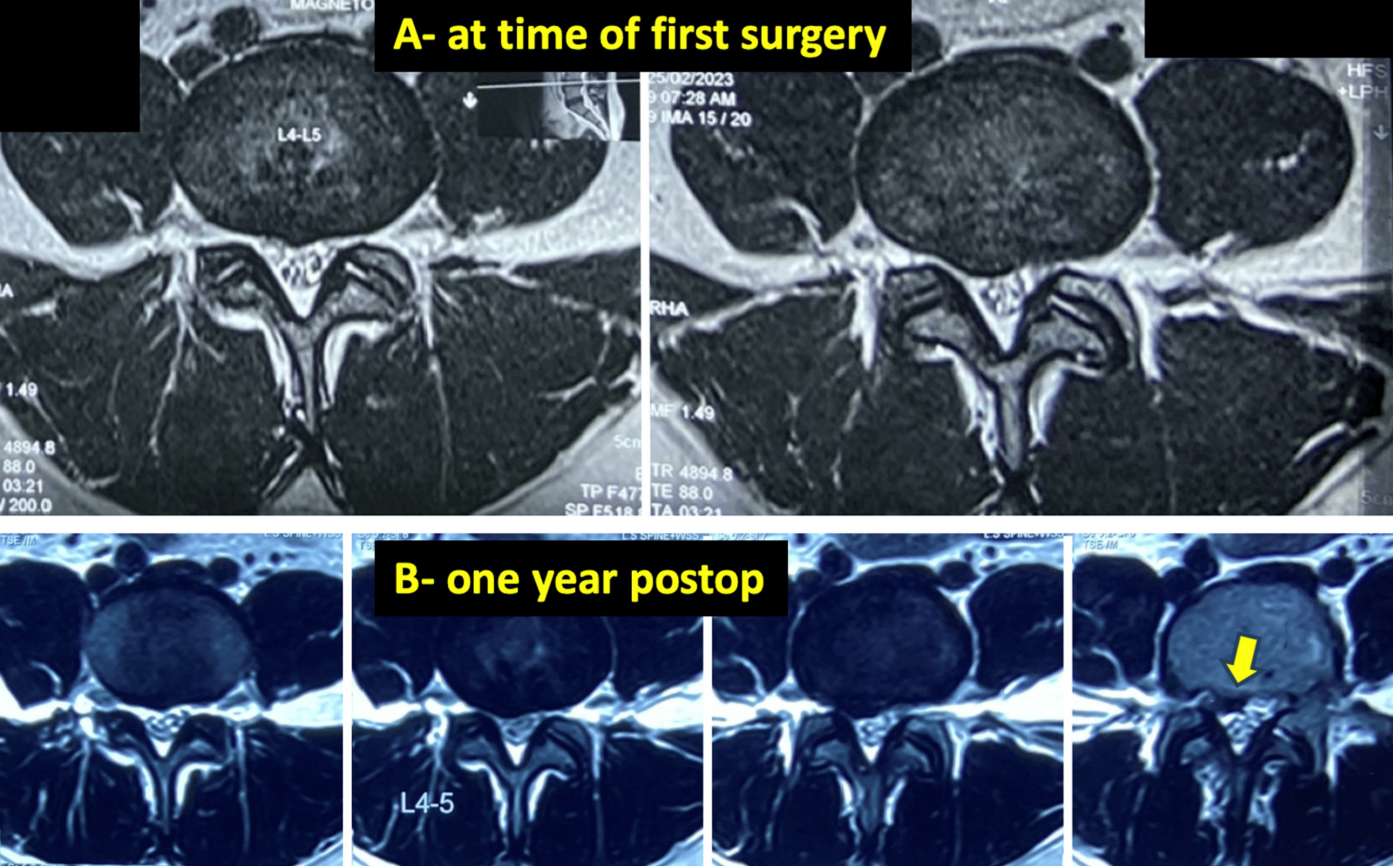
A 33-year-old female patient with recurrent disc requiring revision in a case of postoperative micro lumbar discectomy (MLD) A: preoperative imaging (axial T2 weighted axial MRI)  showing  paracentral disc bulge of L45 level addressed with micro lumbar decompression; B: one year postop imaging (axial T2 weighted axial MRI), showing recurrent disc at the same level which now is down migrated (yellow arrow).
